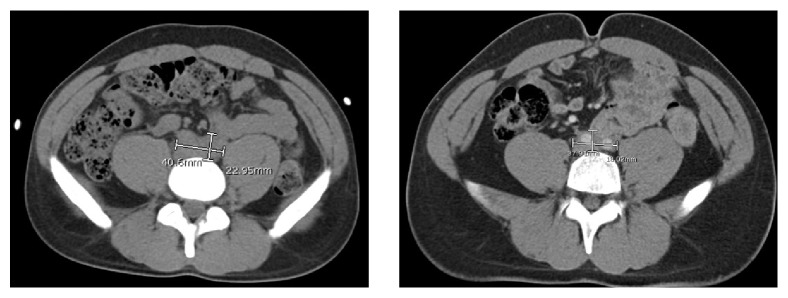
Follow-up abdomen and pelvis computed tomogram with and without intravenous contrast 4 months following a course of corticosteroid therapy revealed significant reduction in the soft tissue mass (approximately 4 cm in diameter compared with 7 cm at presentation).
